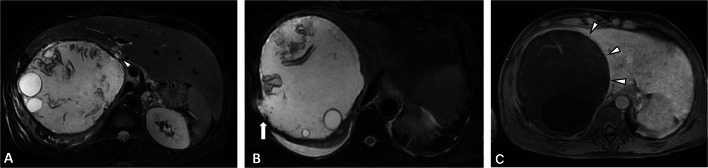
Cystic echinococcosis lesion CE3b in a 48-year-old patient revealed by abdominal pain, fever, and liver blood test abnormalities. A Fat-suppressed T2-weighted MR image in the axial plane shows a cystic lesion of the right liver, including daughter cysts with floating membranes. Biliary compression without fistula can be observed (arrow). B Fat-suppressed T2-weighted MR image in the axial plane. Posterior rupture of the cystic echinococcosis (arrow). C Fat-suppressed T1-weighted sequence in the axial plane shown after gadolinium injection at the portal phase. Parietal enhancement of cystic echinococcosis that suggests infection of parasitic lesion (arrowheads)
